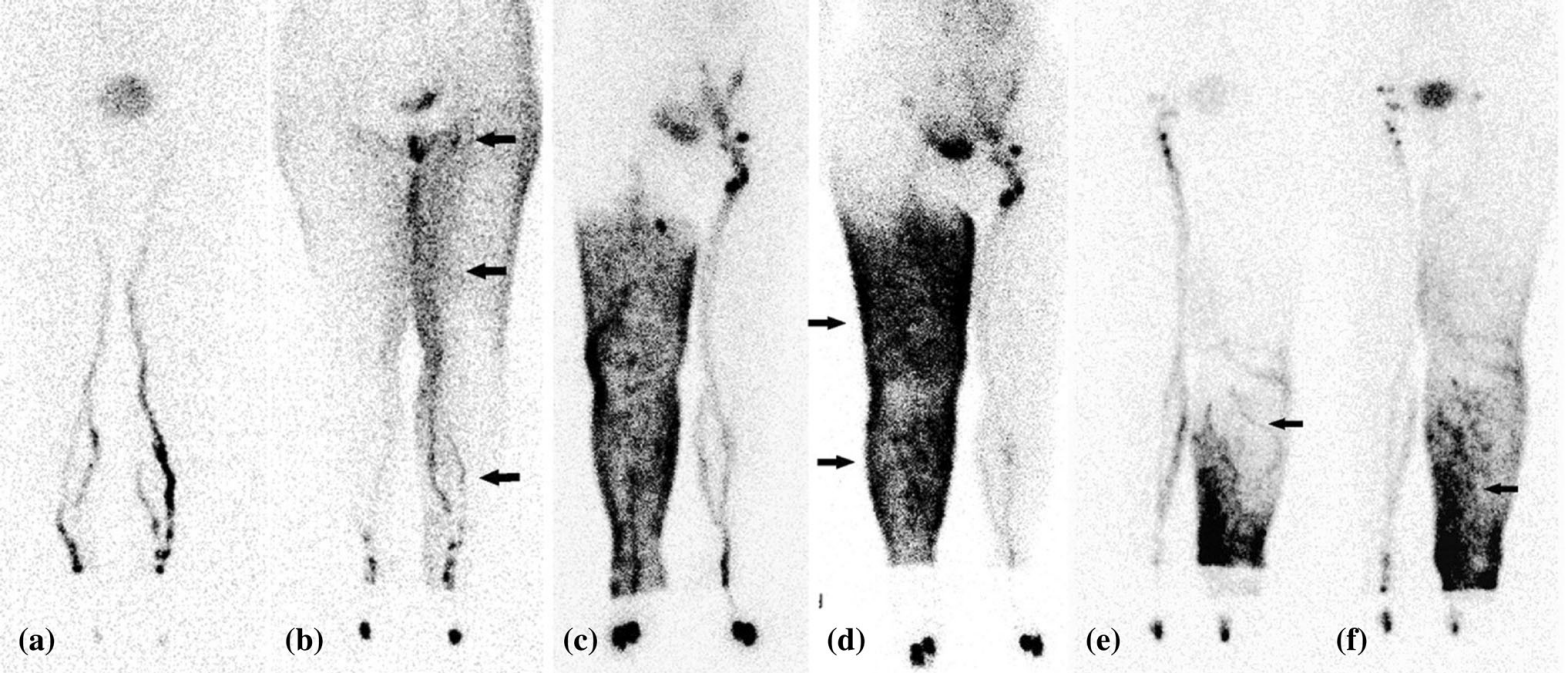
Lymphoscintigraphy imaging of the lymphatics. a, b Images of type II in a patient with left lymphedema a 30 and b 120 min after injection of contrast medium. Lymph stasis in the lymphatics (arrow) and visible dermal backflow (arrow) on the left thigh can be seen. The inguinal lymph nodes are reduced in number (arrow). c, d Images of type III in a patient with right lymphedema c 30 and d 120 min after injection of contrast medium. Dermal backflow (arrows) in the leg and thigh can be seen. e, f Images of type IV in a patient with left lymphedema e 30 and f 120 min after injection of contrast medium. Dermal backflow [arrow in e] and lymph stasis in the lymph vessels [arrow in f] in the leg can be seen and remains in the leg 120 min later. Reprinted from Maegawa et al.^35^ with permission from John Wiley & Sons, Inc
